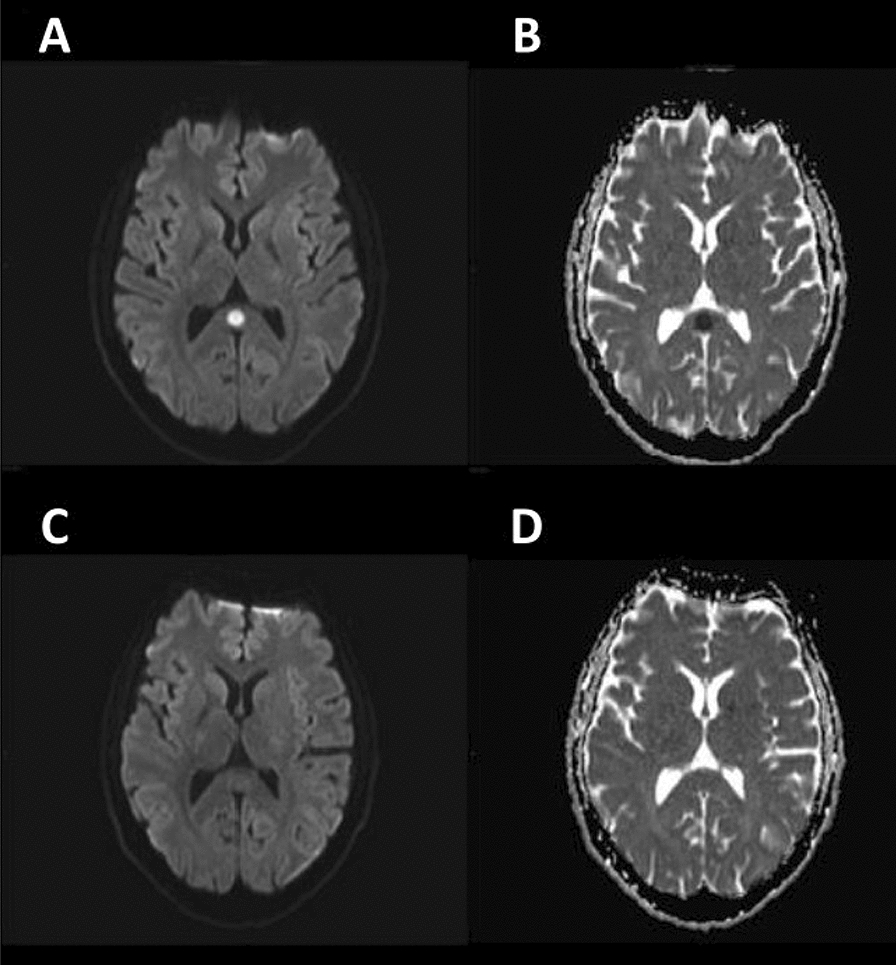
CLOCC. Axial Brain MRI with non-enhancing, midline circular hyperintensity in DWI A and hypointensity in ADC map B in the corpus callosum, suggesting oedema, compatible with CLOCC (“Cytotoxic Lesion Of the Corpus Callosum”). 10 days after, MRI shows complete resolution of the lesion C and D
